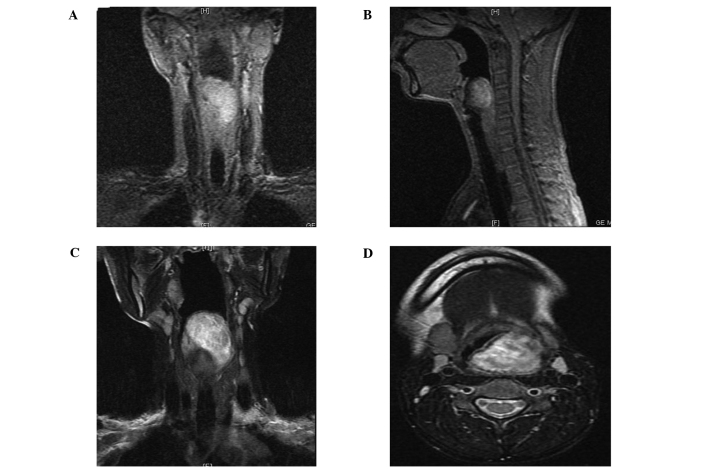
MRI scans from a 29-year-old female. T1-weighted (A) coronal section of the larynx and (B) sagittal section of the larynx. T2-weighted (C) coronal section of the larynx and (D) axial section of the larynx. MRI, magnetic resonance imaging.
